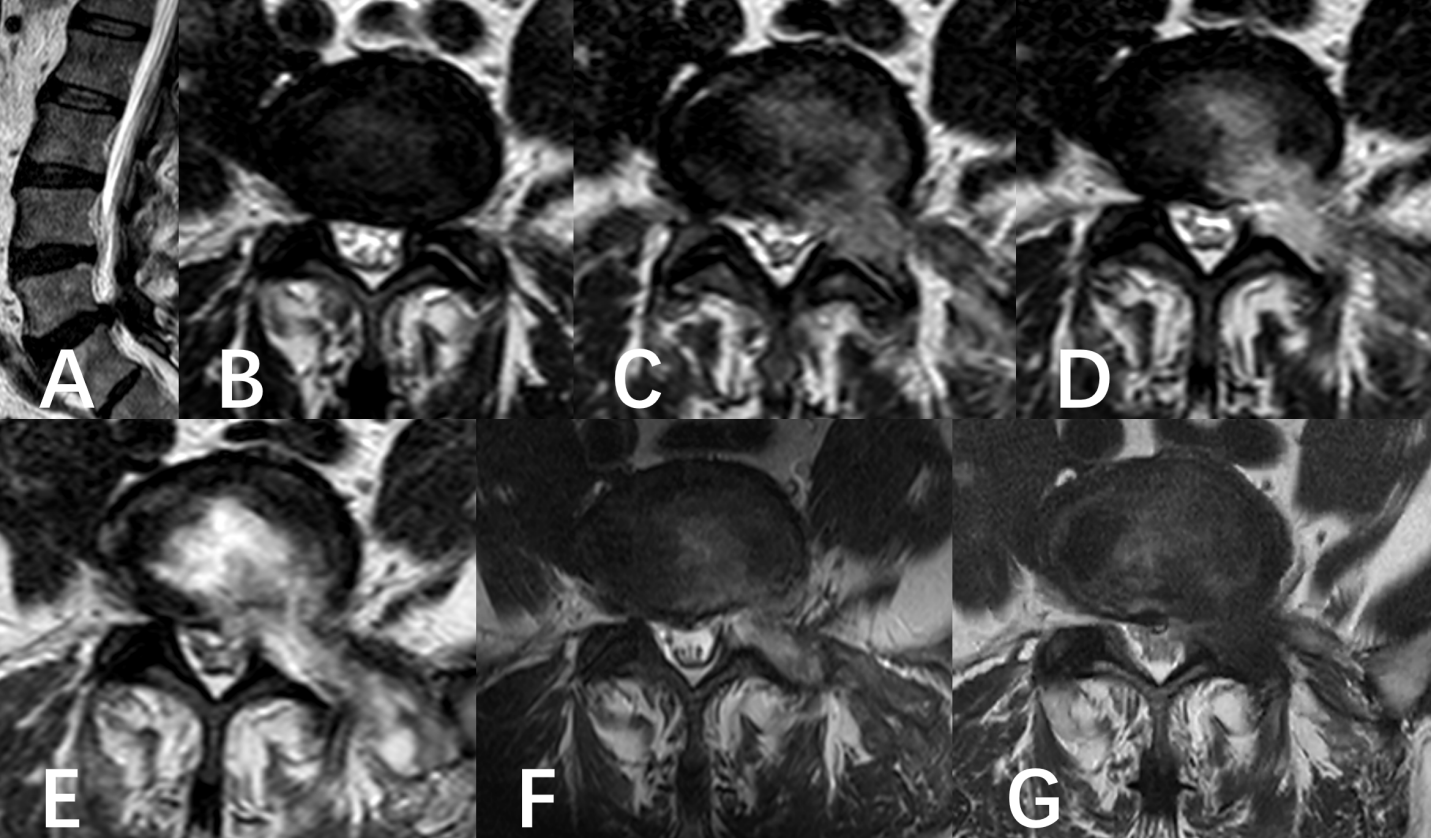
A six-year-old male was diagnosed with L5/S1 lumbar disc herniation and underwent PED. (A, B) Preoperative MR showed the left disc herniation before operation. (C–F) Follow-up of postoperative MRI images. (C) showed the state of the operation area immediately after operation. (D) was the state of operation area at the beginning of anti-infection treatment. (E) was the state of the operating area near the most serious period of infection captured in the anti-infection process. (F) was the result of the last follow-up before discharge. (G) was the result of 6 months follow-up.
